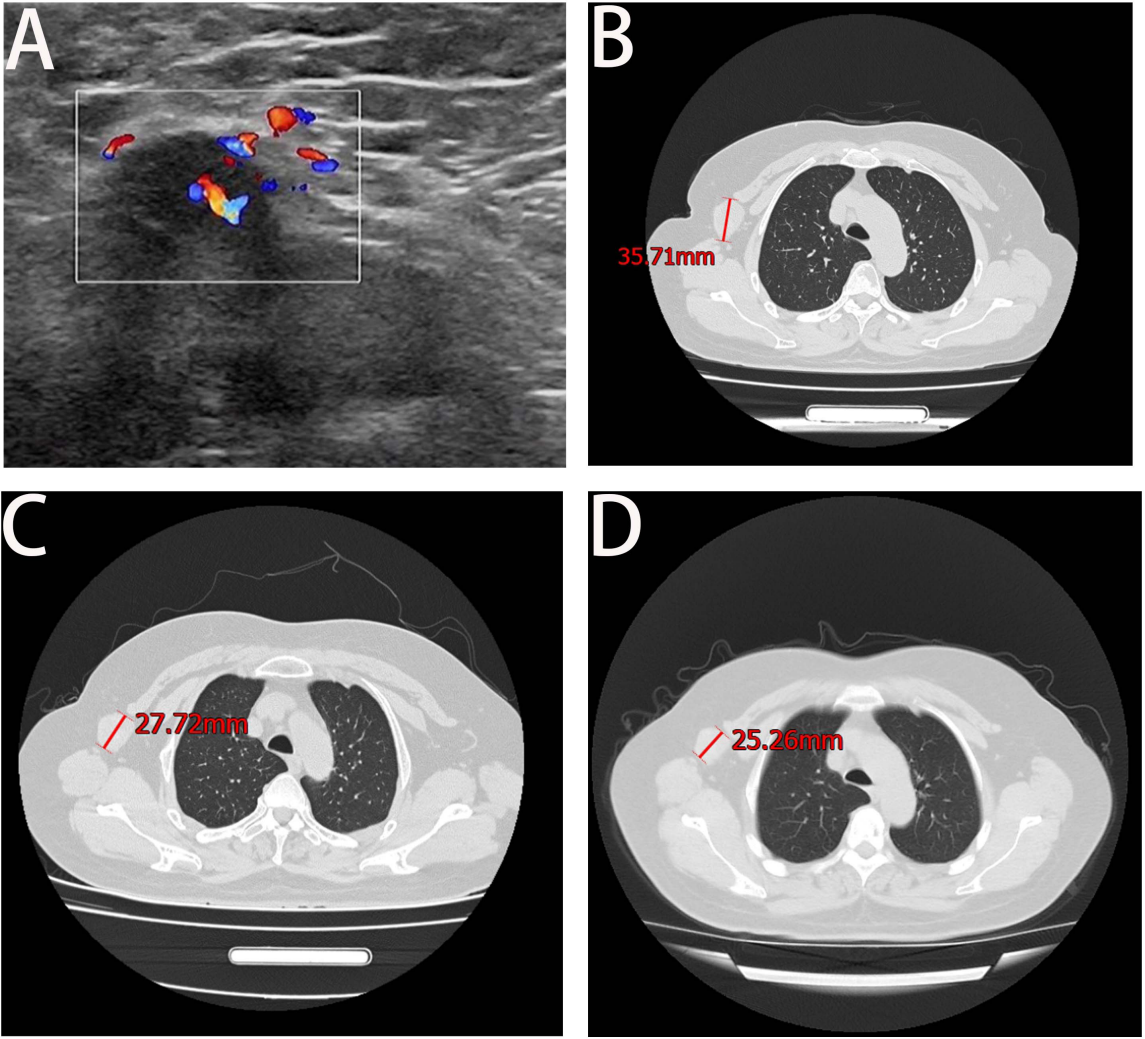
Axillary ultrasound and lung CT. Panel (A) shows the right axillary probe and irregularly shaped hypoechoic mass, measuring approximately 32 mm × 26 mm. Panel (B) shows the maximum axillary mass diameter at the time of initial admission. Panel (C) shows a significant reduction in the maximum diameter of the irregular soft tissue mass under the right axilla at the end of the entire chemotherapy cycle. Panel (D) displays the result of the February 2024 review, which indicated a decrease of approximately 2 cm in the maximum tumor diameter from the previous review.
